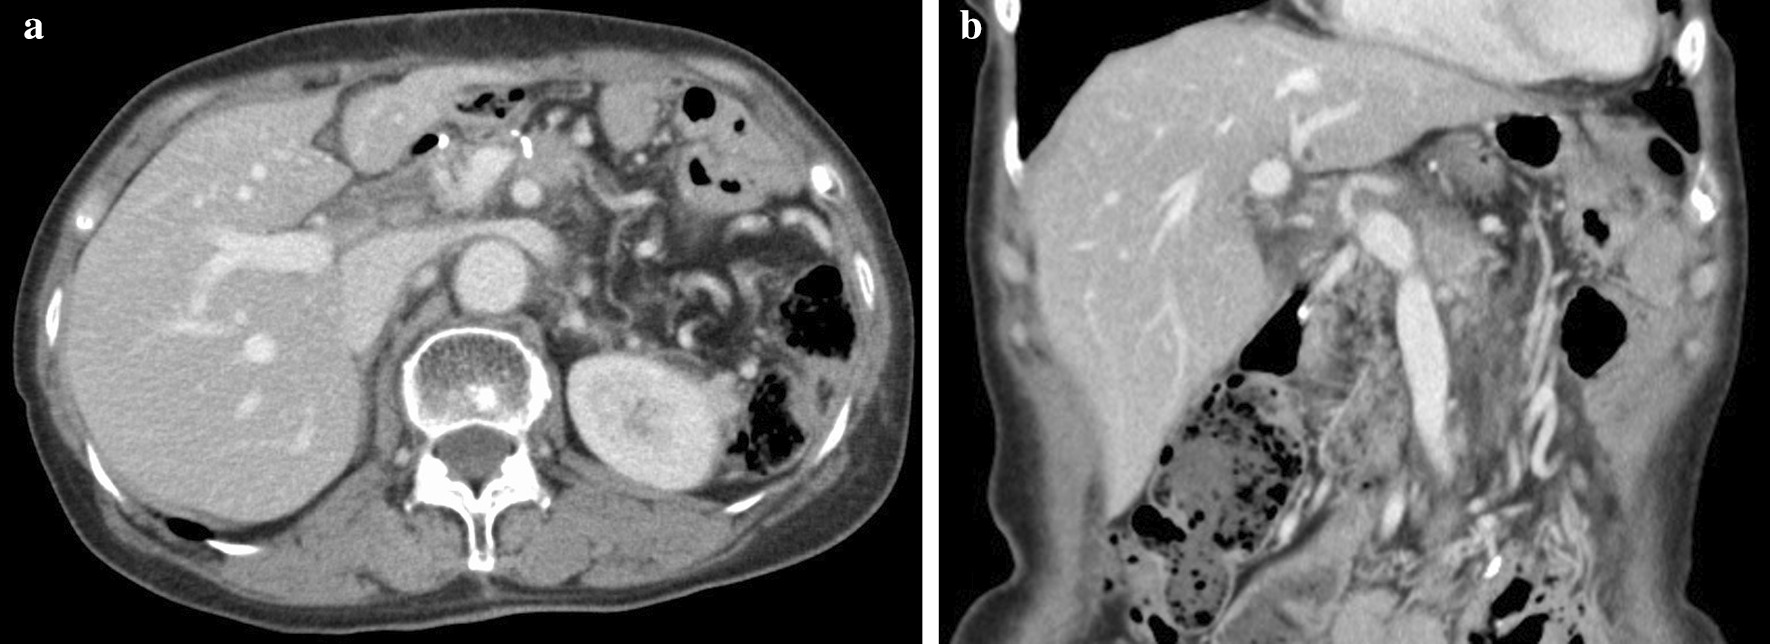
a Computed tomography (coronal plane). A nodule image measuring approximately 13 mm is visible. b Computed tomography (axial view of the same location as in Fig. 5-1)
